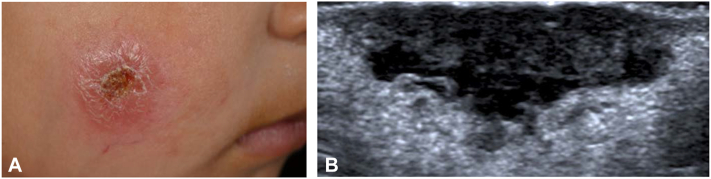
Cutaneous leishmaniasis lesion caused by a member of the *Leishmania donovani/infantum* complex on the right cheek of a 3-year-old girl. A, Ulcerated, erythematous plaque sized 3 cm in diameter with raised borders and central crusting. B, Ultrasound image showing a well-defined, inhomogeneous, hypoechoic subcutaneous lesion measuring 2 × 1 cm on the right cheek.
